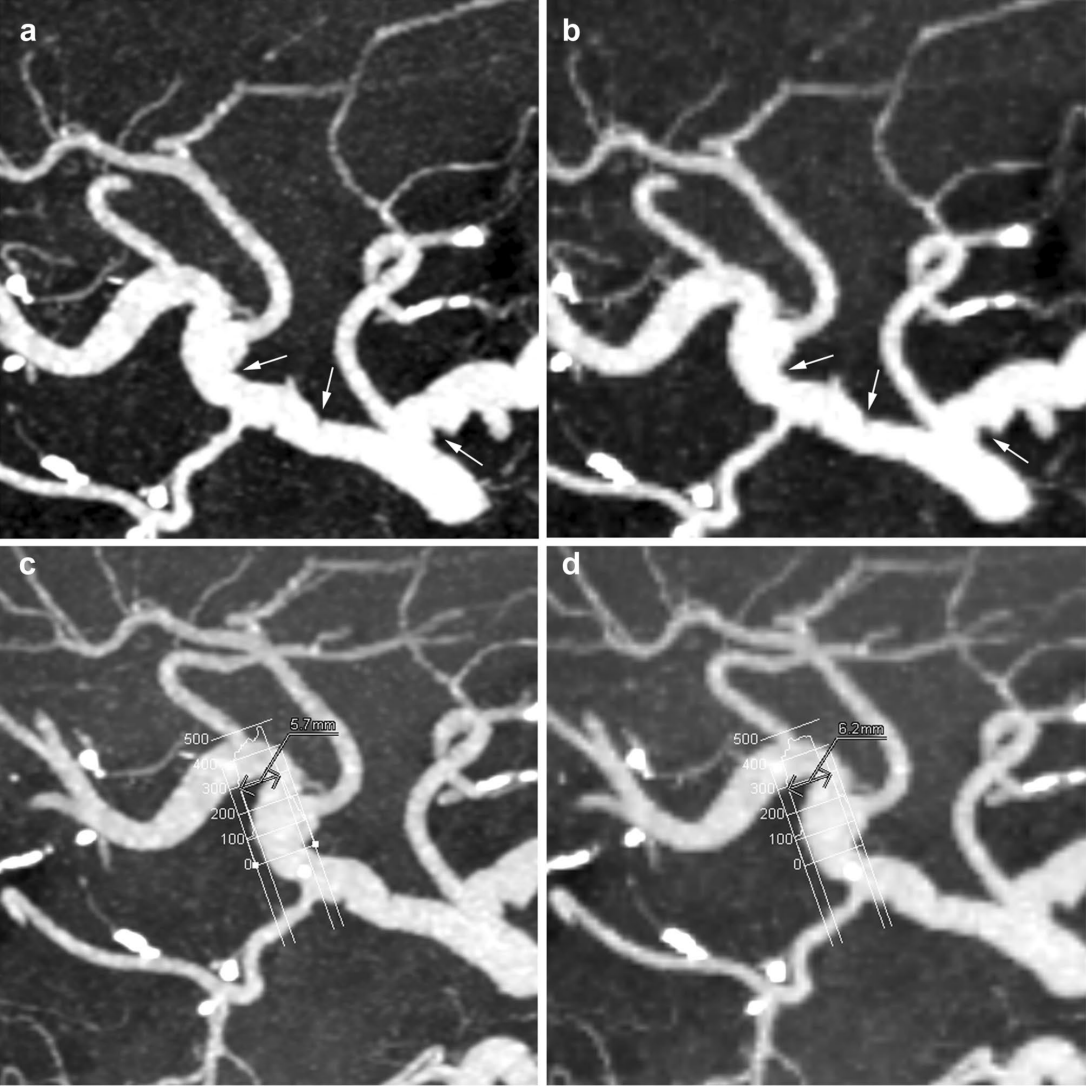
Partial MIP image of CTA of branches of the celiac axis. a: HR CTA with a matrix of 1024 × 1024 more clearly showed caliber irregularities of the common hepatic artery, splenic artery, and PHA (arrows) due to the encasement of pancreatic cancer than (b): NR CTA with a matrix of 512 × 512. Profile curves of the PHA of (c): HR CTA and d: NR CTA
